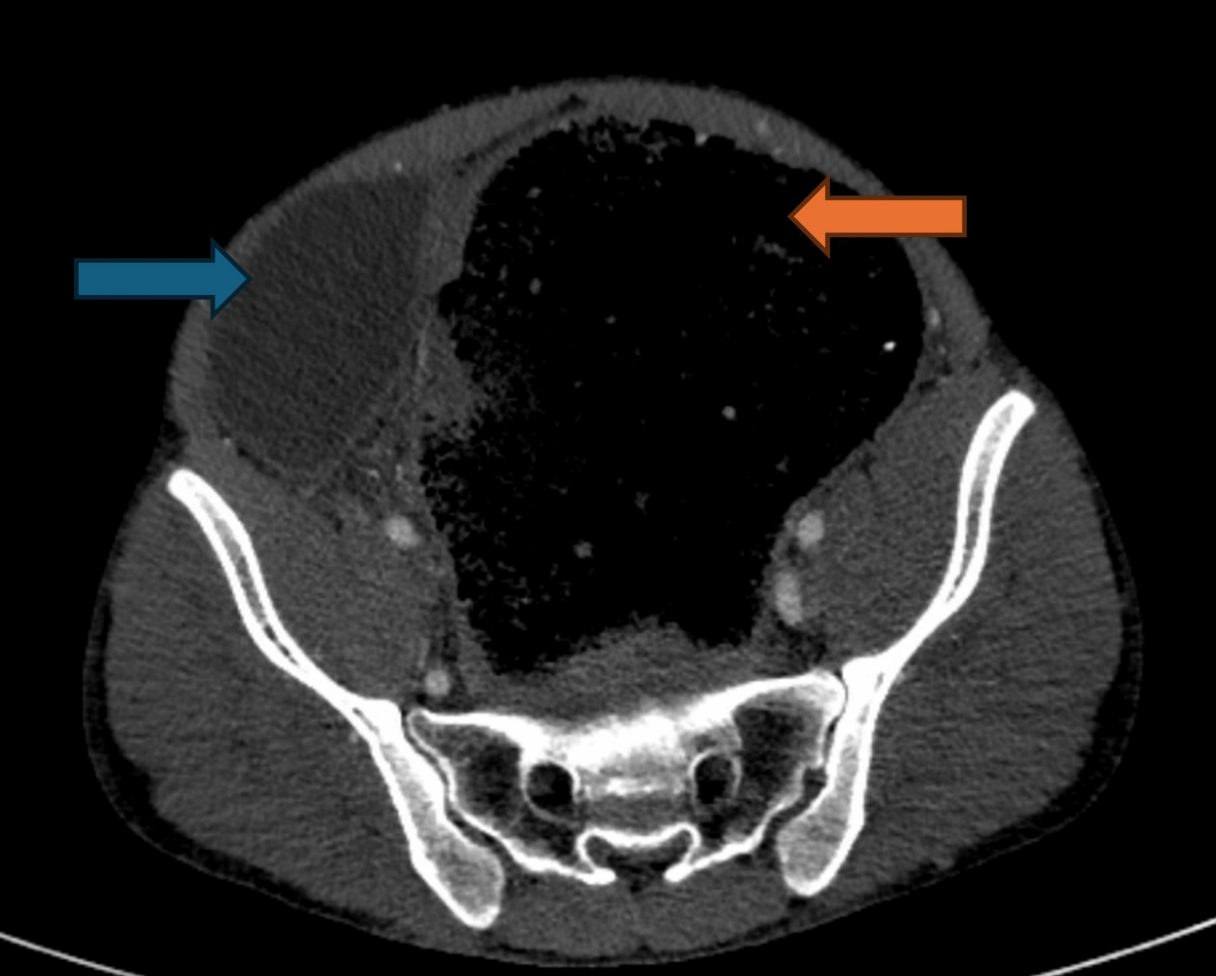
CT axial view of pelvis showing large intestinal gas burden (orange arrow) with displacement of the urinary bladder to the right (blue arrow)
